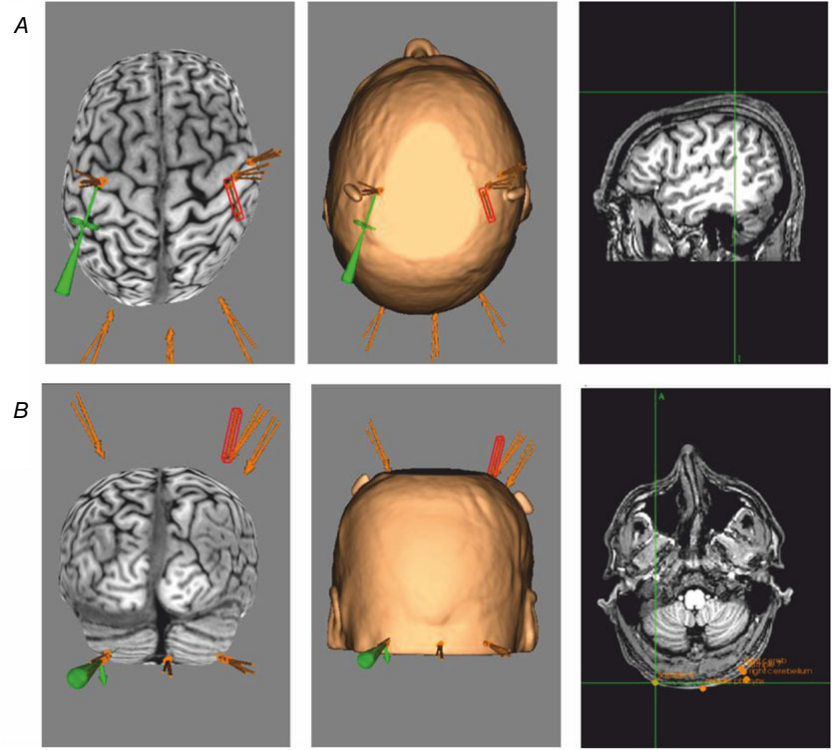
Cortical and cerebellar stimulation points from a participant's MRI using frameless functional MRI stereotaxy (Brainsight 2) [Color figure can be viewed at wileyonlinelibrary.com]
